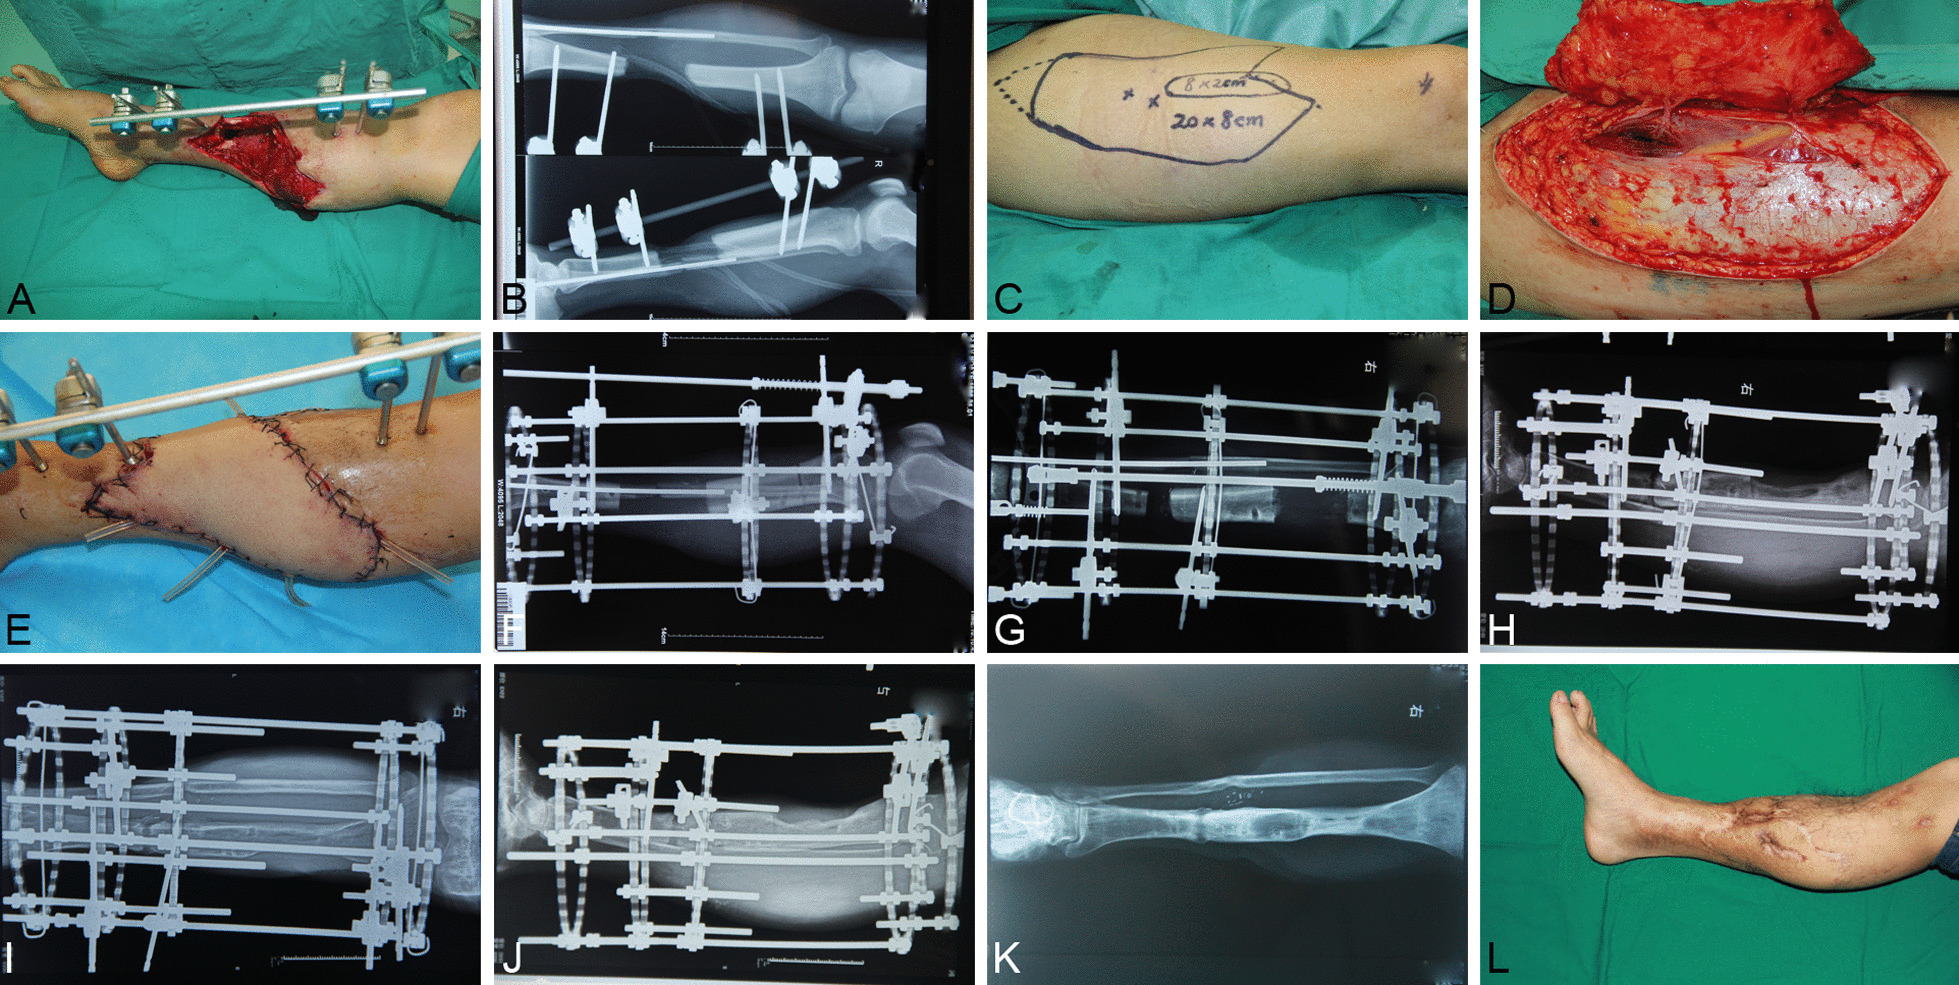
A 26-year-old male presented with an open tibia and fibula fracture due to traffic injury. A The right leg after radical debridement. B Preoperative X-ray, C The designed ALTP flap of donor site. D Free ALTF was harvested. E Postoperative view of ALTF transplantation. F X-ray after Ilizarov bone transport. G–J Postoperative radiographic view at the 3-month, 12-month, 18-month, and 24-month follow up. K Postoperative follow-up was 30 months, and X-ray films were taken 1 month after removal of the external fixator. L Postoperative view of the affected limb 30 months after surgery
